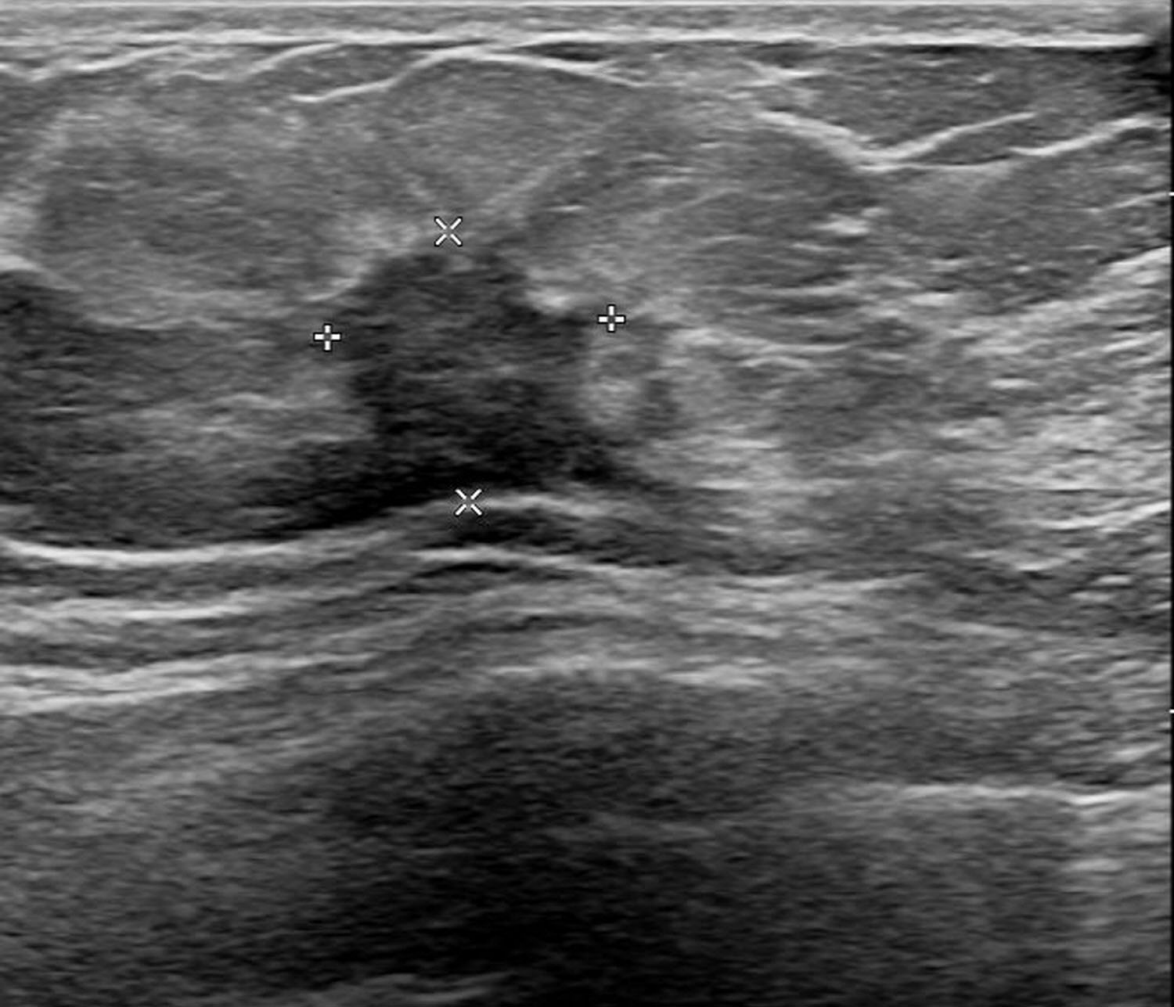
Ultrasound image of a breast lesion.
